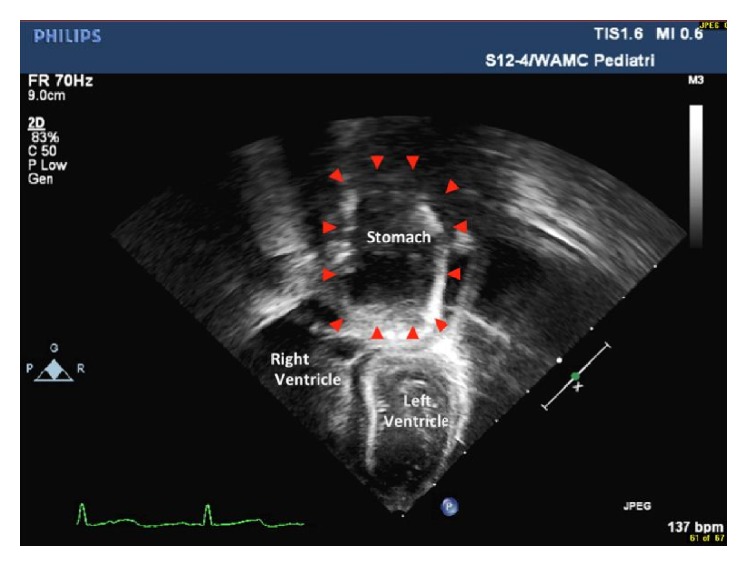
Modified apical 4-chamber view (posterior angulation) showing the stomach (arrowhead) above the left ventricle in the thorax outside of the pericardium.
